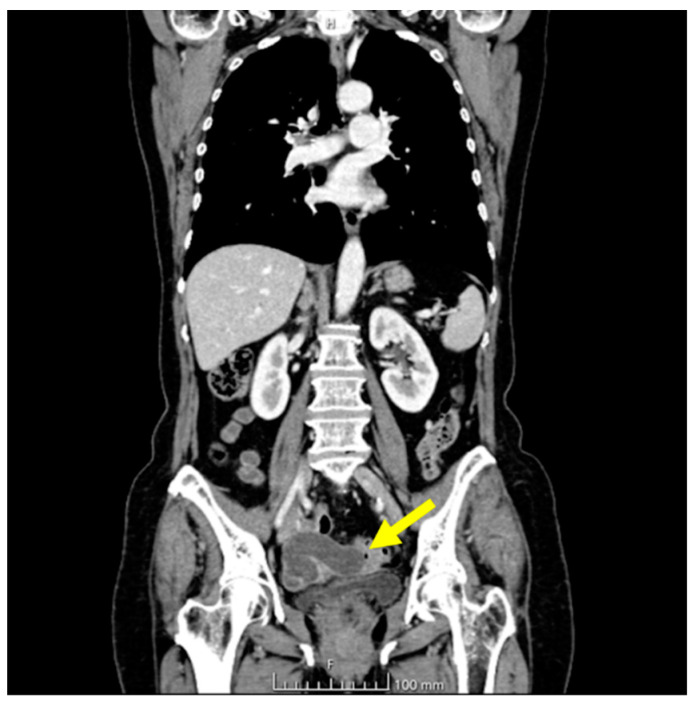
CT chest and abdomen with contrast, portal phase. The yellow arrow indicates the tubal lesion.
